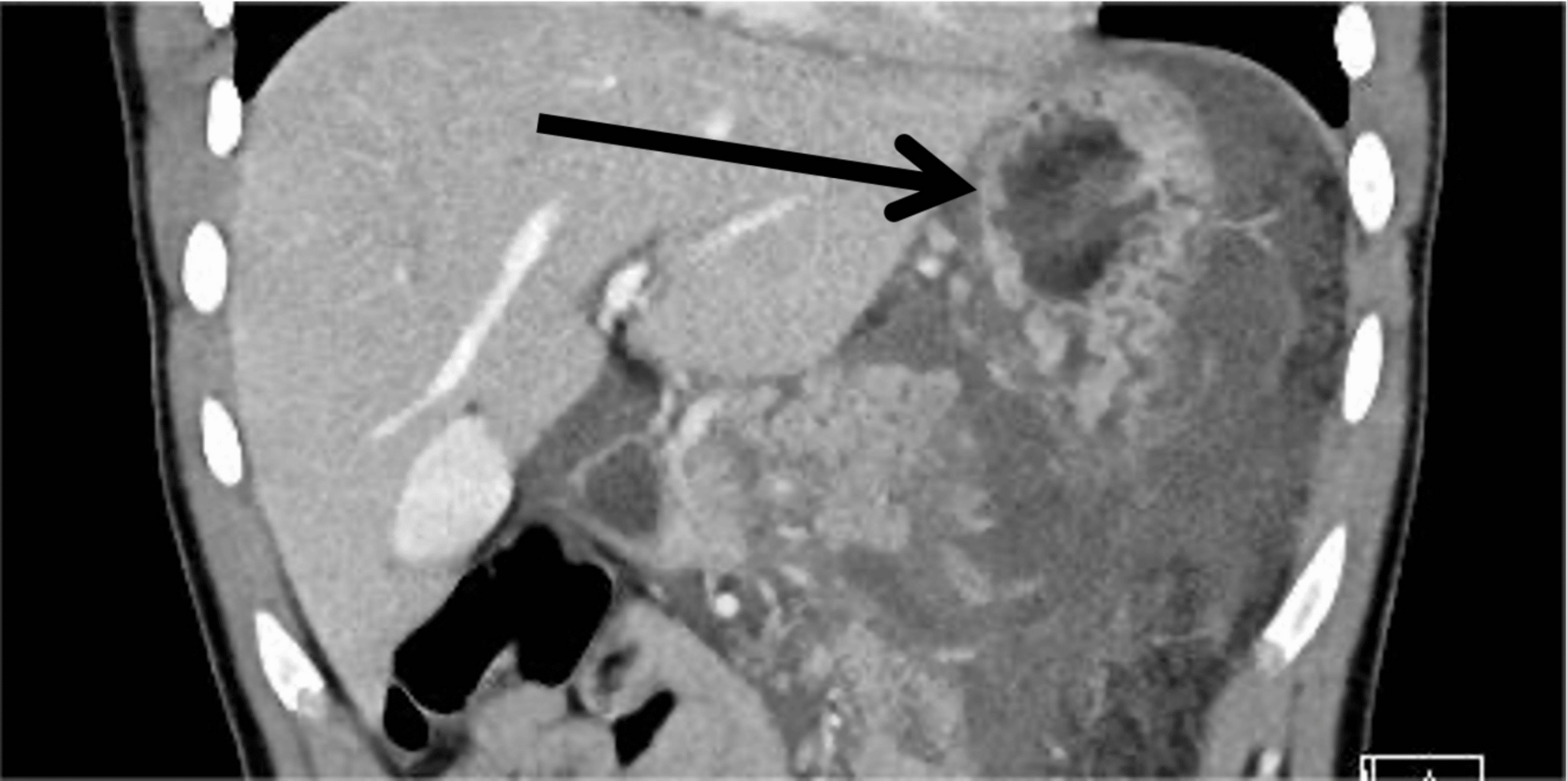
Contrast-enhanced CT scan (coronal view) The black arrow indicates the transection of the distal pancreas. CT: computed tomography
